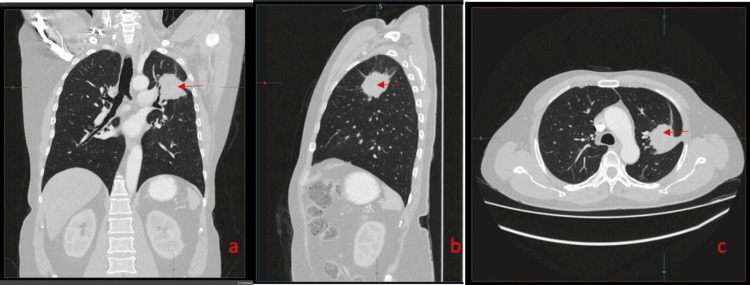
CECT images of the lung showing the tumour in (a) coronal section, (b) sagittal section, and (c) transverse section (red arrows). CECT: contrast-enhanced computed tomography
